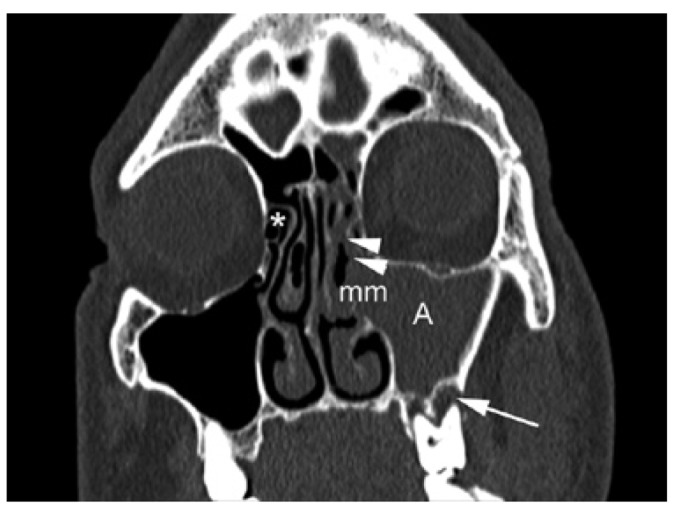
CT scan (coronal plane) corresponding to a 49 years old man patient showing a left odontogenic maxillary sinusitis extended to anterior ethmoid sinus. There are a diffuse opacification of the maxillary sinus (A) and anterior ethmoid sinus (arrowheads). The middle meatus (mm), where are situated the ostium of maxillary and anterior ethmoid sinus, is compromised by the inflammatory process. The anterior ethmoid sinuses on the right side are normal (*). The arrow indicates the location of a periapical lesion (abscess) adjacent to the sinus floor.
